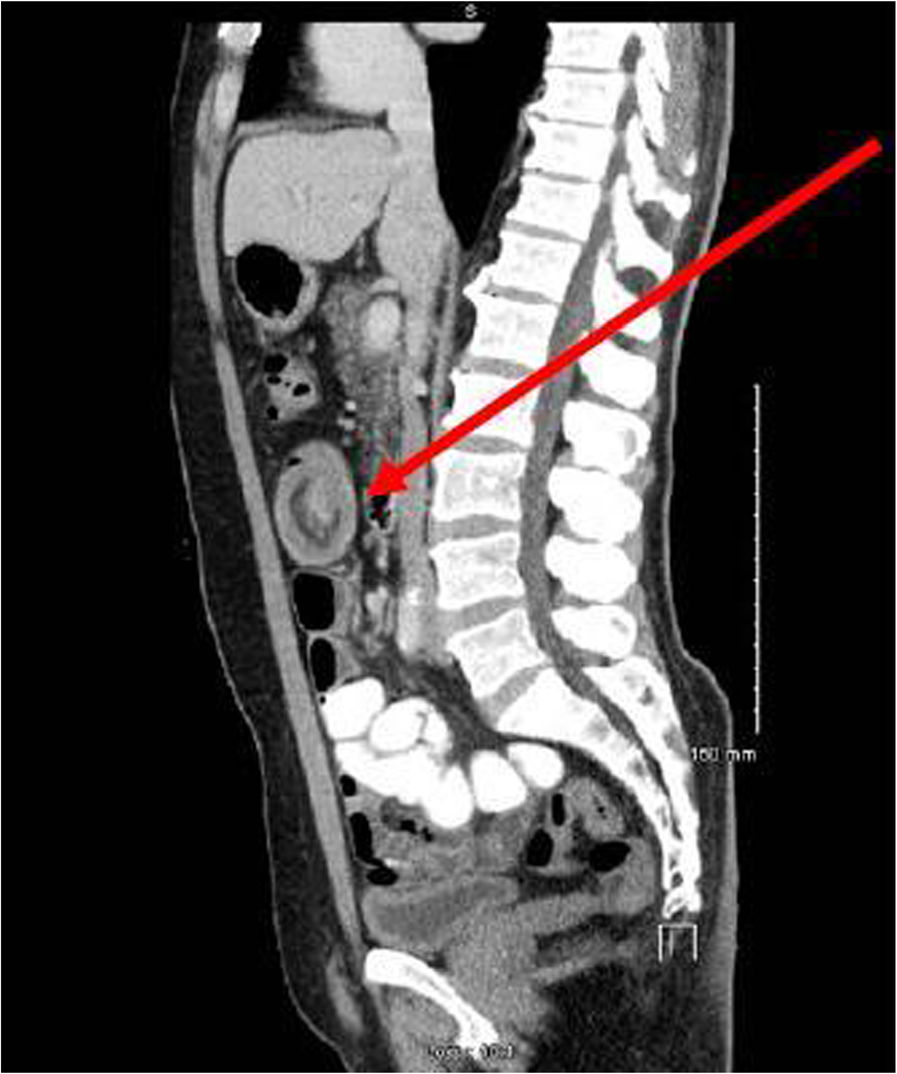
MRI showing jejunal intussusception (red arrow).
